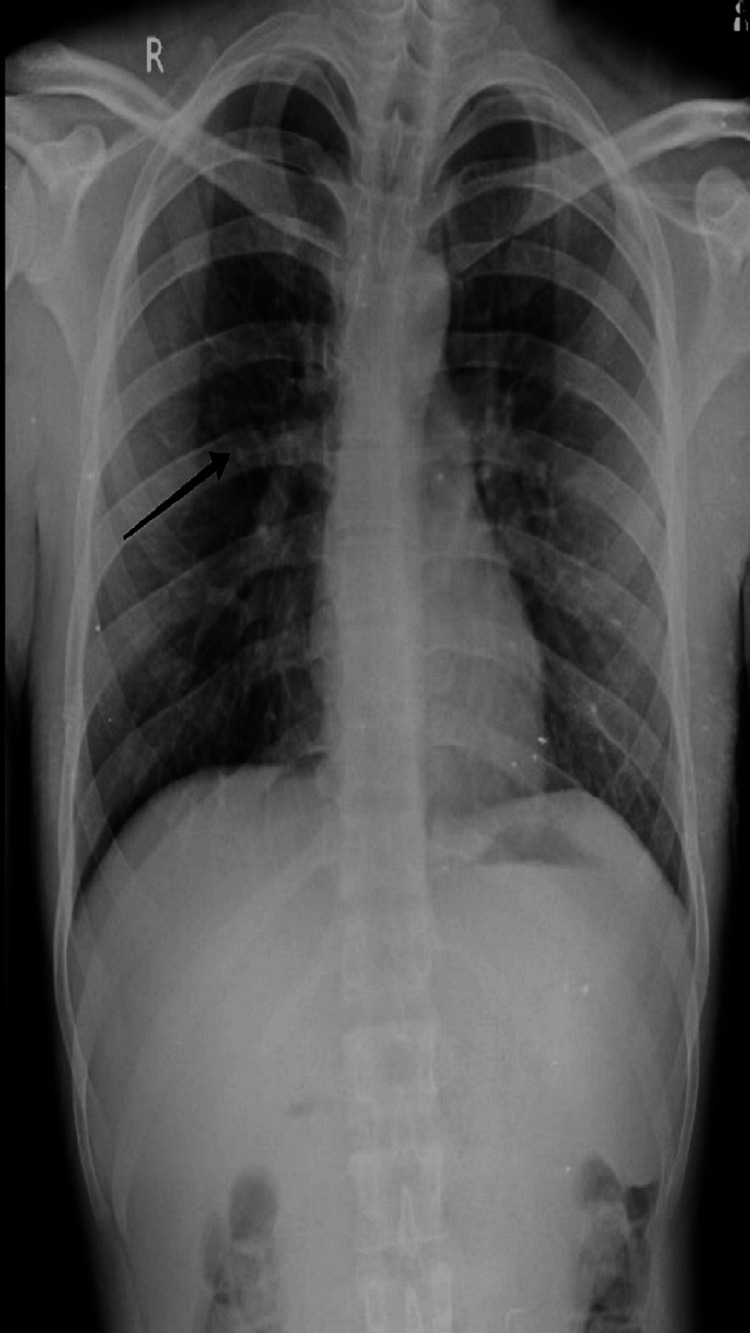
Chest X-ray showing pneumonia infection
